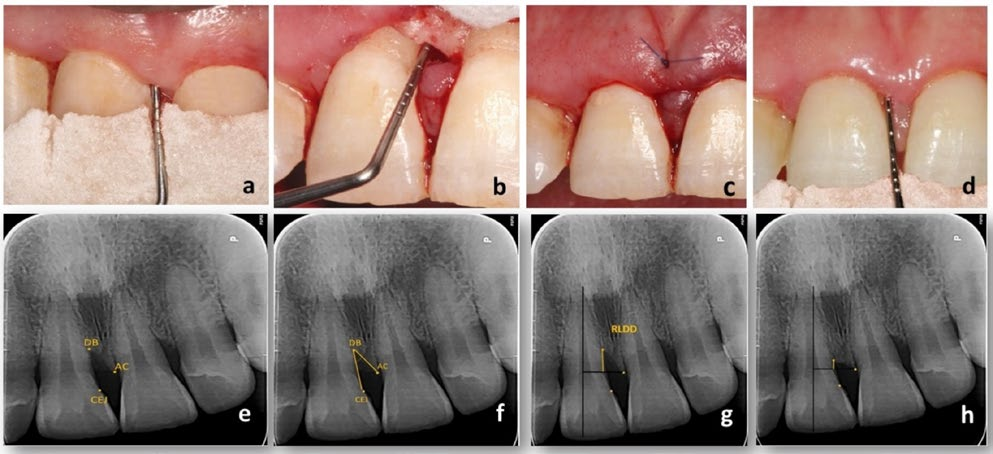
(a) Preoperative clinical photograph showing acrylic stent fabricated for standardization of the clinical measurements (b) Clinical photograph showing de‐granulated intra‐bony defect and confirming the depth ≥ 3 mm. (c) Clinical photograph showing the internal vertical mattress suture done using 6/0 Polypropylene (d) Clinical photograph showing probing depth after 9‐month follow‐up. (e) Periapical radiograph for the upper right central incisor showing three reference points: Alveolar crest (AC), defect base (DB) and cemento‐enamel junction (CEJ). (f) Periapical radiograph for the upper right central incisor showing the radiographic defect angle (RDA) of the mesial intraosseous defect formed between the three reference points. (g) Periapical radiograph for the upper right central incisor identifying reference lines: A vertical line corresponding to the long axis of the tooth and a horizontal perpendicular line passing through AC and identifying Radiographic linear defect depth (RLDD) of the pre‐treatment calculations. RLDD was measured as the depth of the intraosseous defect from the horizontal line passing through the AC to the DB. (h) Periapical radiograph for the upper right central incisor identifying RLDD of the post‐treatment calculations.
